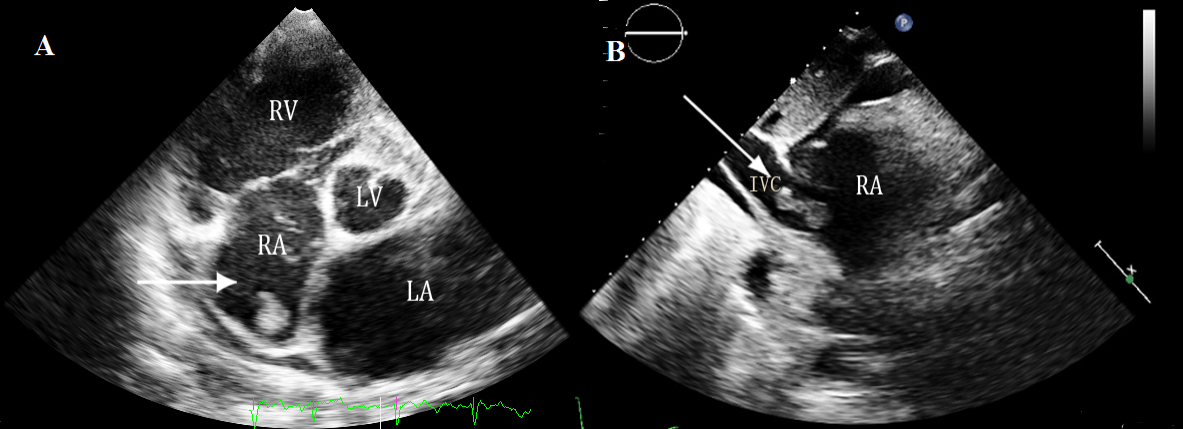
A mass of right atrium: A) Parasternal short axis view by 2D TTE, B) Subxyphoid view by 2D TTE.
